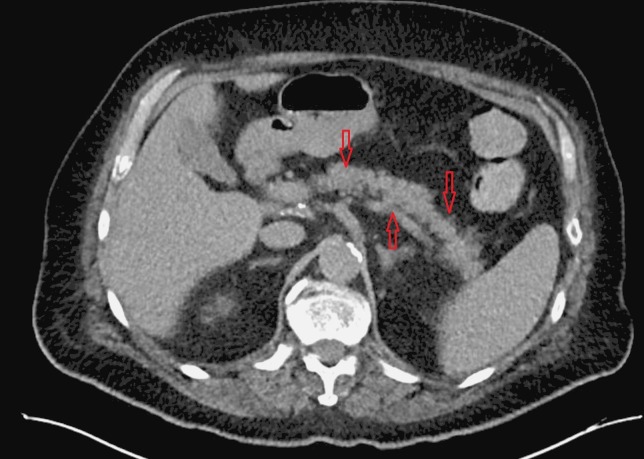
Normal pancreas. CT-angiography (CTA) of abdomen demonstrating normal pancreas (red arrows). Note the distinct border without any enlargement.
